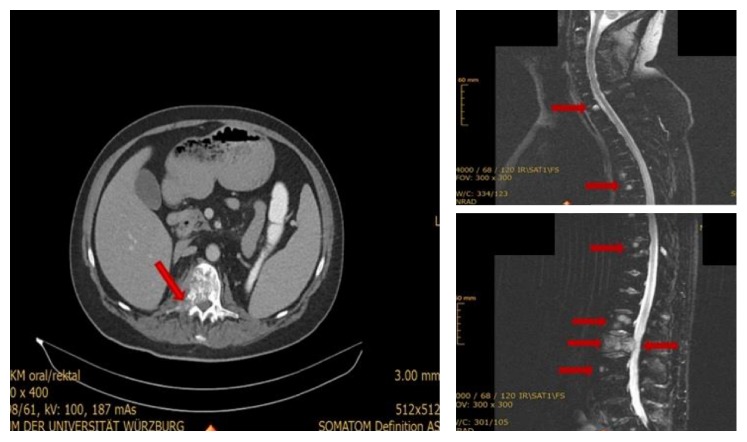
MRI and CT findings at diagnosis showing a pathologic facture at the right side of the first and second lumbar vertebral body from a metastatic solid mass, as well as multiple osteolytic metastases from 7th cervical to 2nd lumbar vertebra (arrows).
